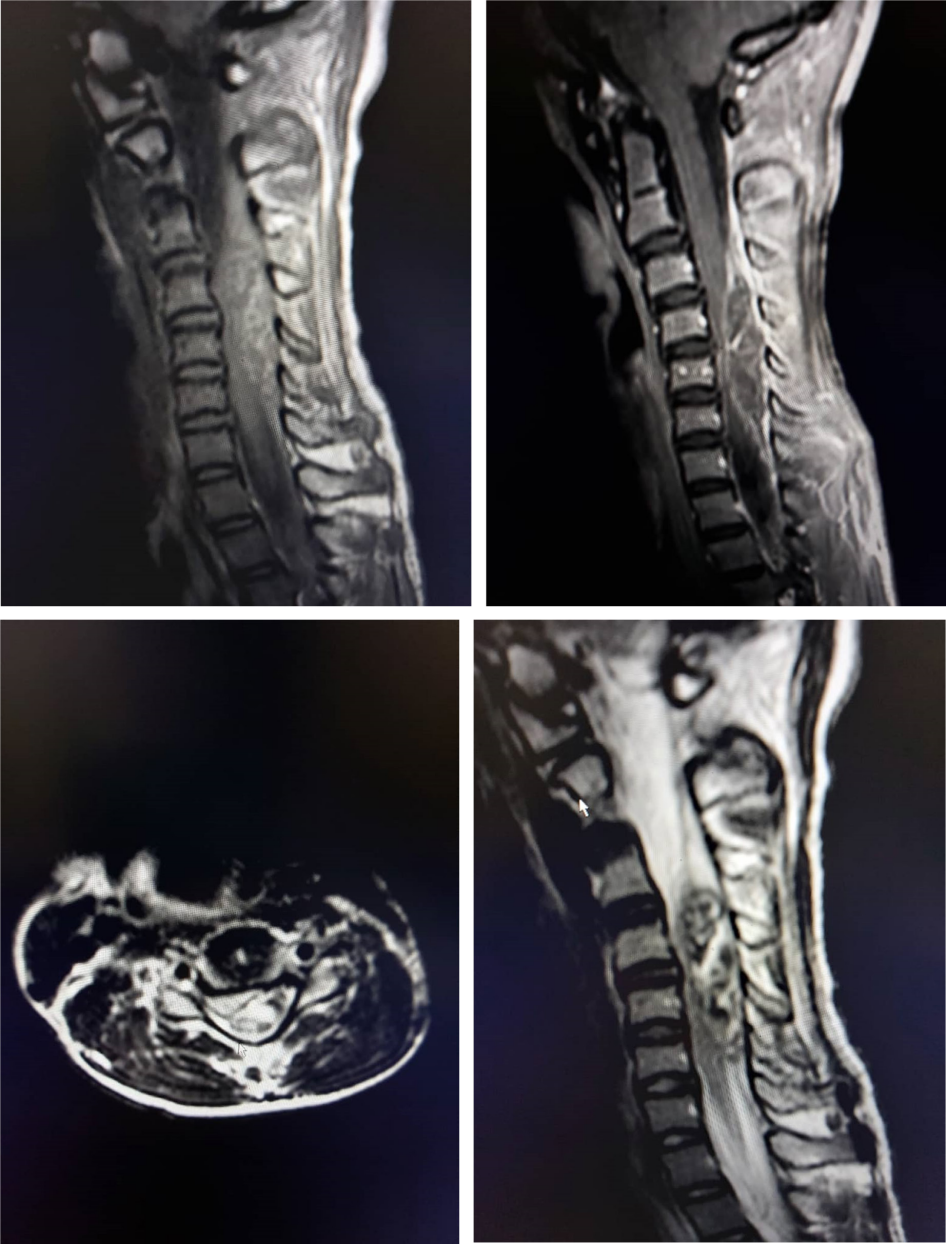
Magnetic resonance imaging of the patient with spontaneous idiopathic spinal subdural hemorrhage; in sagittal T1 images, mixed and hyper‐signal intensity lesion and in T2 images mixed and hypo‐signal lesion are seen. After gadolinium injection, only mild peripheral enhancement was seen. In axial T2 images, the lesion is located posterolateral to the spinal cord in the left side.
